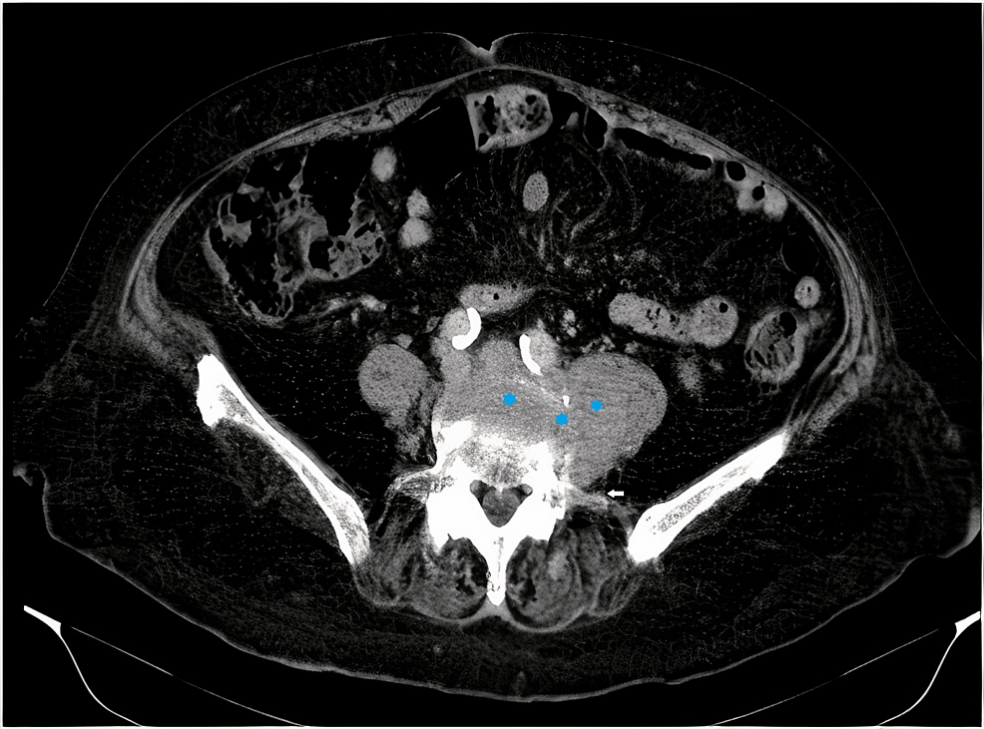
Computed tomography scan of the lumbar spine. Soft tissue mass that infiltrates the L4 vertebral body (stars), the pedicle, and left transverse apophysis (arrow), extending to the L3-L4 and L4-L5 intersomatic spaces and also intracanal extension.
